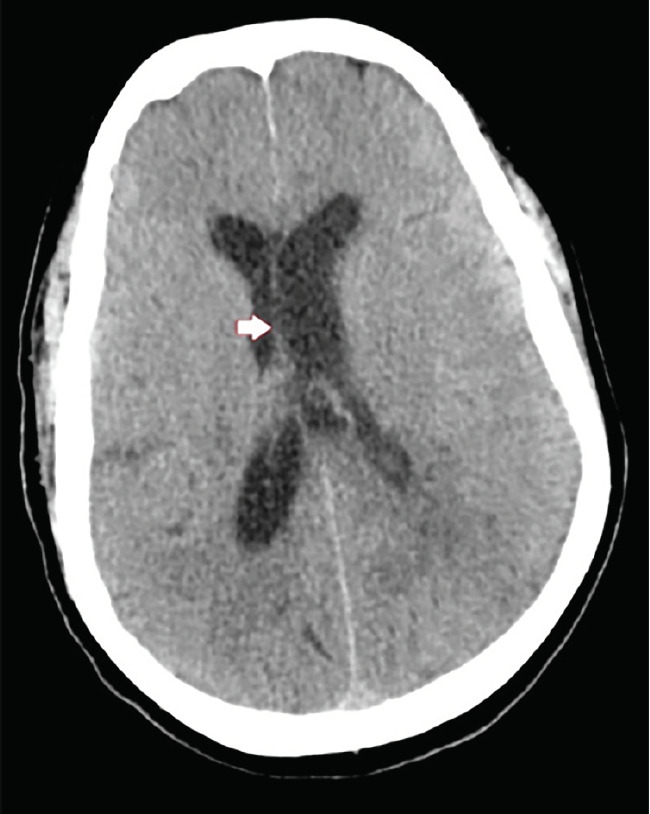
Axial computed tomography of the head with intravenous contrast showing mild enlargement of the left lateral ventricle (arrow).
